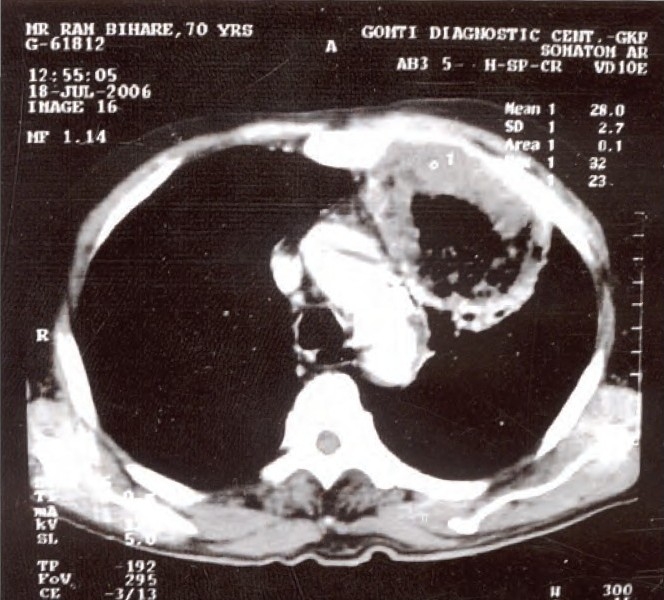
Computed tomography revealed large thick walled cavity on left side in left upper lobe abutting chest wall and encroaching towards arch of aorta.
